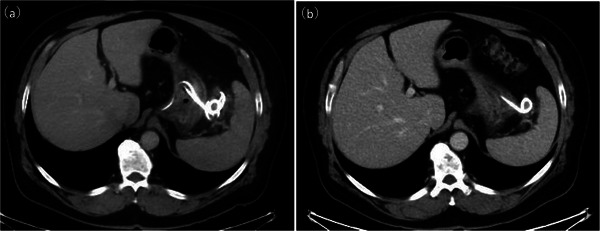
(a) CT image obtained 1 week after EUS‐guided drainage, showing a reduction in the size of the abscess cavity. (b) CT image obtained 1 month after EUS‐guided drainage, showing near‐complete resolution of the abscess cavity. CT, computed tomography; EUS, endoscopic ultrasound.
